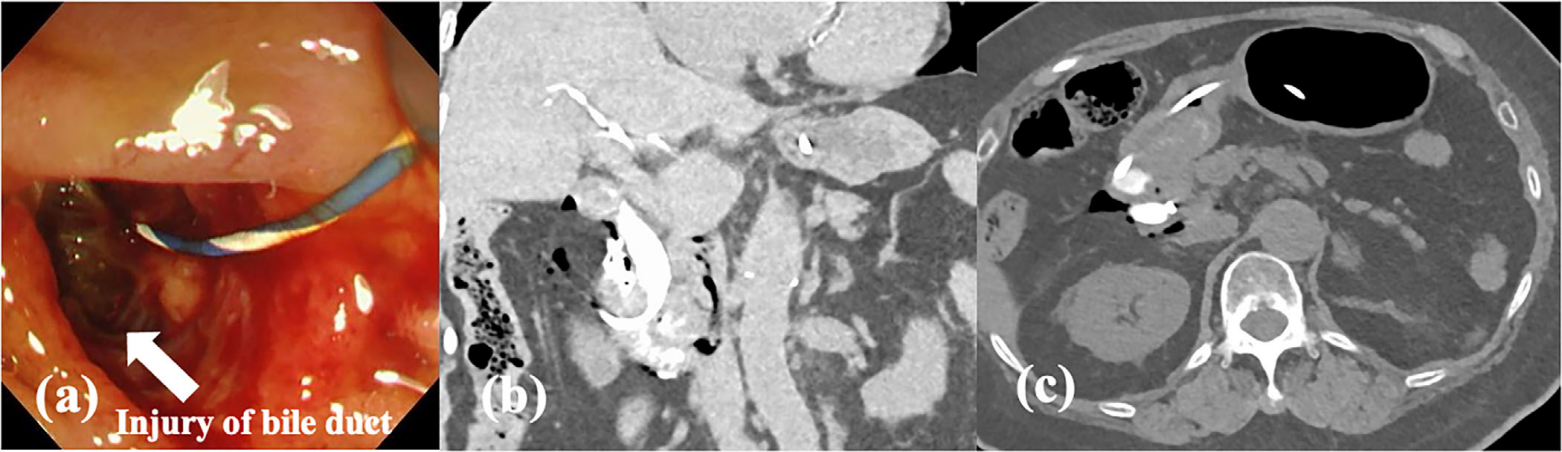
(a) Endoscopic view after endoscopic papillary large balloon dilatation showed injuries to the bile duct and hepatoduodenal mesentery from the posterior wall of the bile duct. (b, c) Computed tomography after endoscopic retrograde cholangiopancreatography showed perforation and accumulation of contrast medium at the posterior wall of the descending region of the duodenum, and free air from around the duodenum to the right retroperitoneum.
